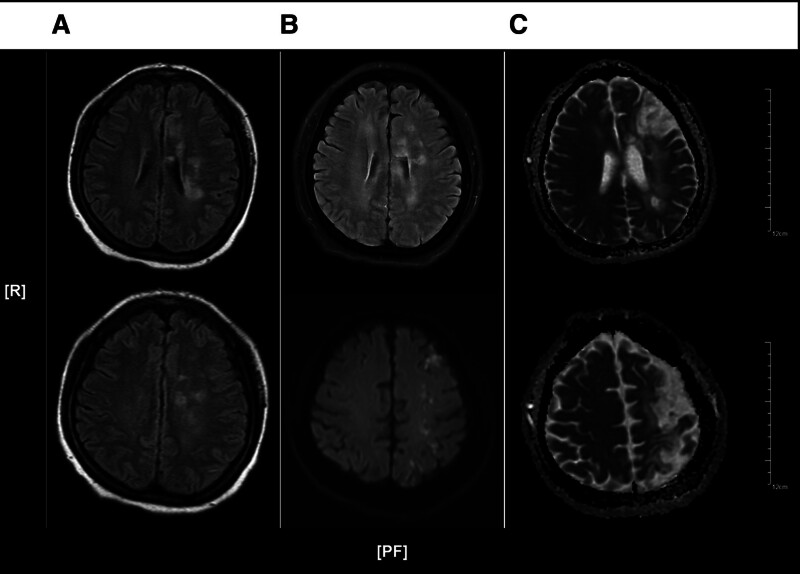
Representative brain magnetic resonance imaging (MRI) scans from Patient 1 during 3 separate admissions, demonstrating progressive cerebral infarction characterized by newly developed ischemic lesions at each hospitalization: (A) first admission, (B) second admission, and (C) third admission.
